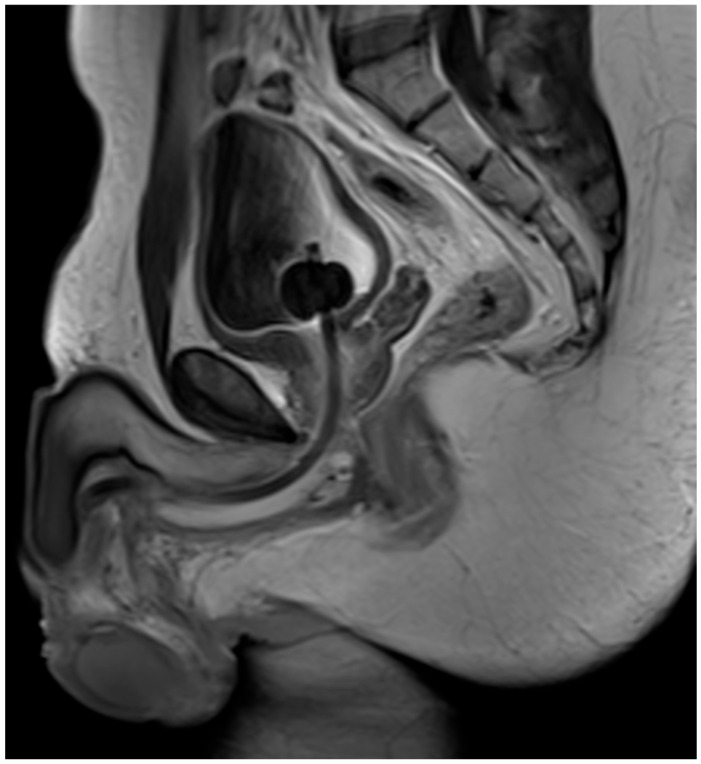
Follow-up MRI found no evidence of tumor recurrence, dehiscence, or fistula.
